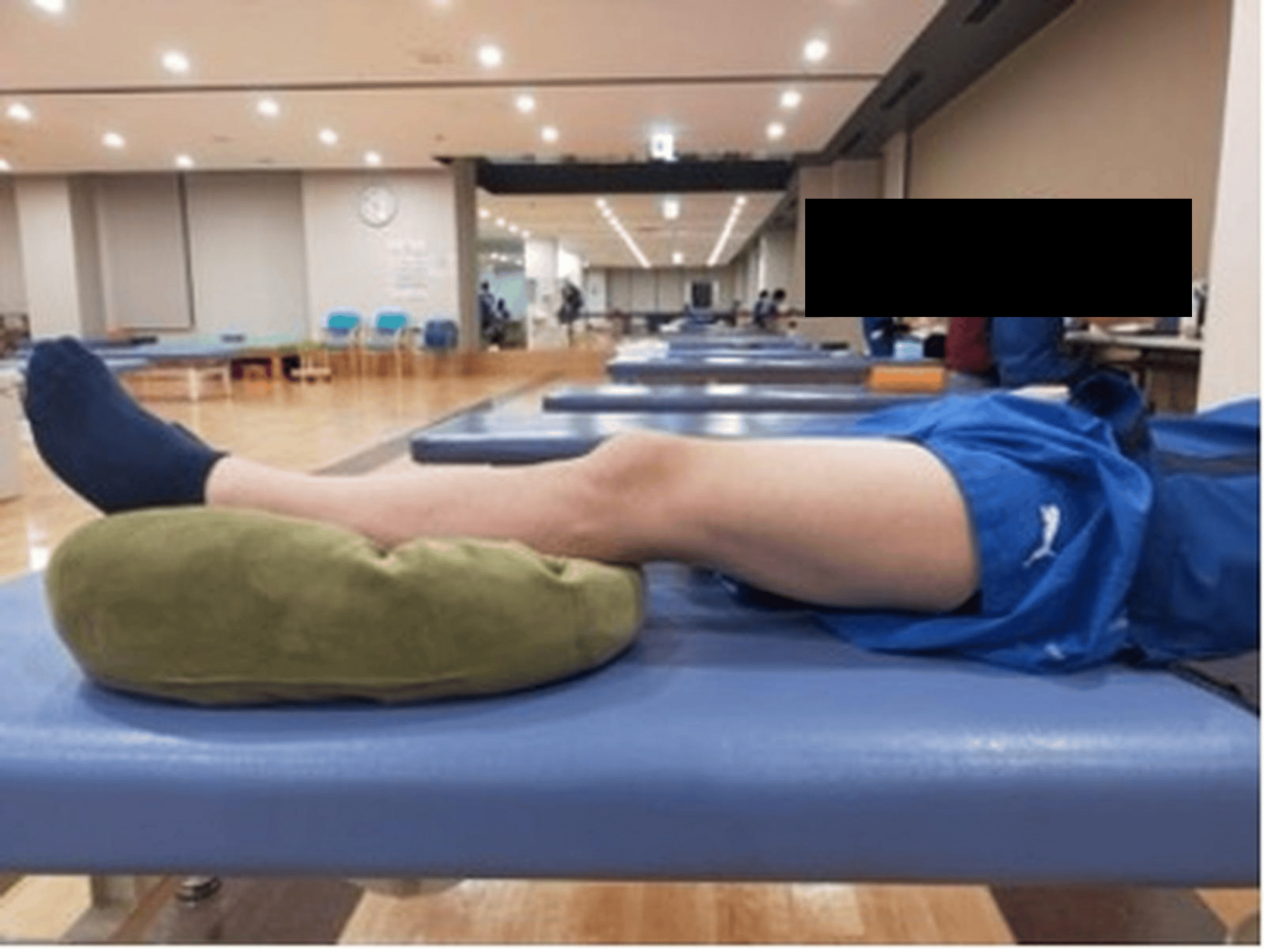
Ultrasound examination positioning Patients were positioned in a relaxed supine position with cushions placed distally to both knees, keeping the knees extended and the lower limbs in a neutral position.
